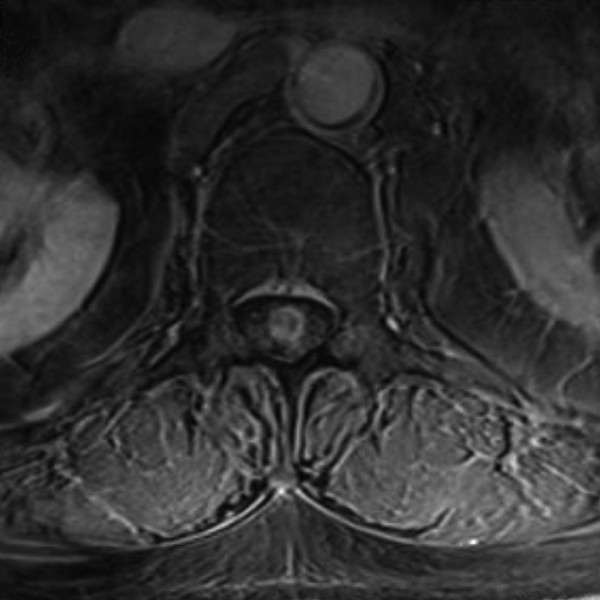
Axial contrast-enhanced T1-weighted magnetic resonance image.
